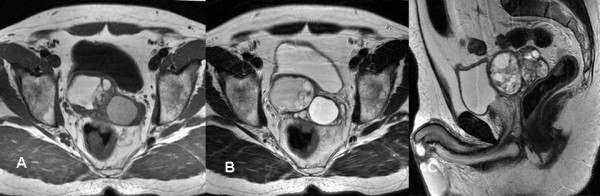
Axial spin-echo T1-weighted (a) and axial (b) and sagittal T2-weighted (c) MRI showed a large, well-defined, multilocular pelvic mass in the side of the seminal vesicles, contiguous to the posterior wall of the urinary bladder and the prostate. On T1 and T2-weighted images the mass showed internal septations that delimited heterogeneous iso- hyperintense areas with proteinaceus content.
